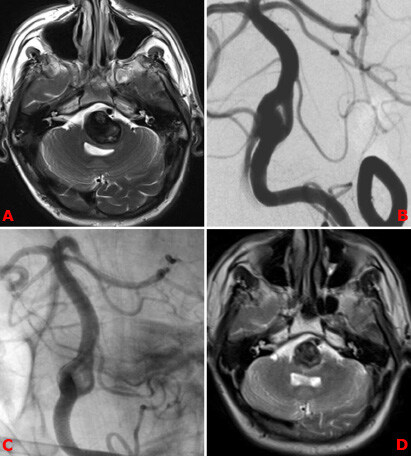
Fifty-year-old male patient presented with respiratory depression. A complex proximal basilar artery aneurysm related with BAF compressing the pons is seen on magnetic resonance imaging (MRI) (A) and DSA (B). It was treated with flow diverter stenting (C). MRI control after 1 month. Pons compression was regressed (D).
